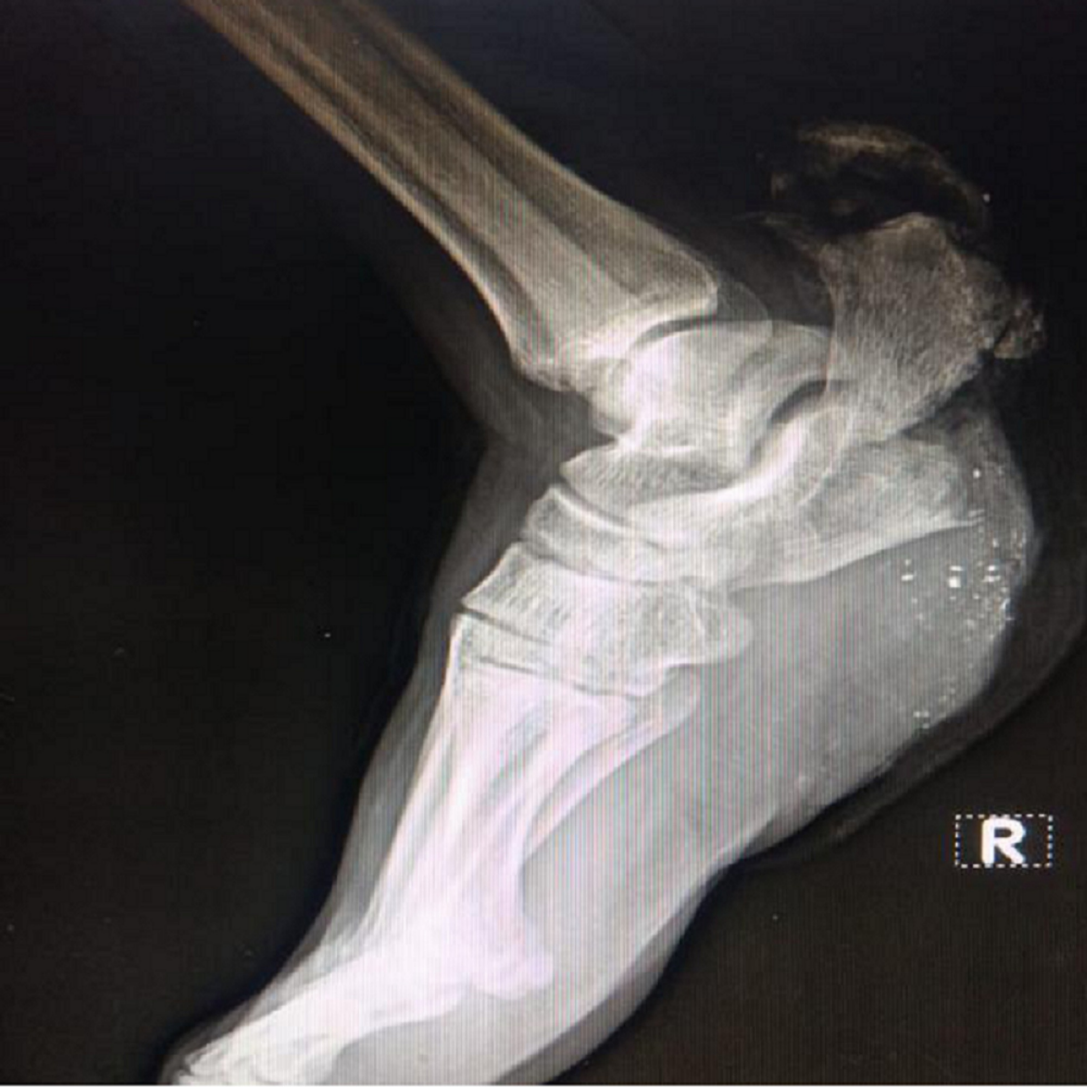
Plain radiographs of the right foot lateral view showing comminuted right calcaneum fracture with bone loss.
